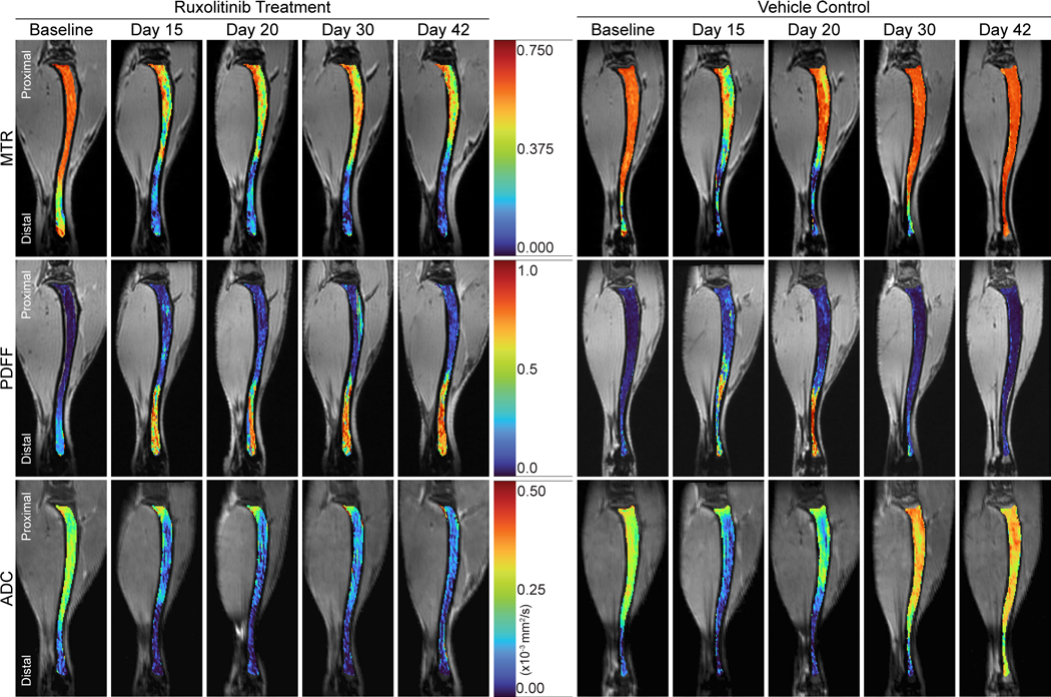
Quantitative MRI metrics show regional variations in disease progression and during treatment. Representative, pseudocolored ablation-only and healthy BMT sagittal BM slices of MTR, PDFF, and ADC overlaid on corresponding, time point–matched, nonquantitative, grayscale background images (MToff; multi-gradient multi-echo mean; and low b-value). Pseudocolor scales depict range of values for each parameter.
